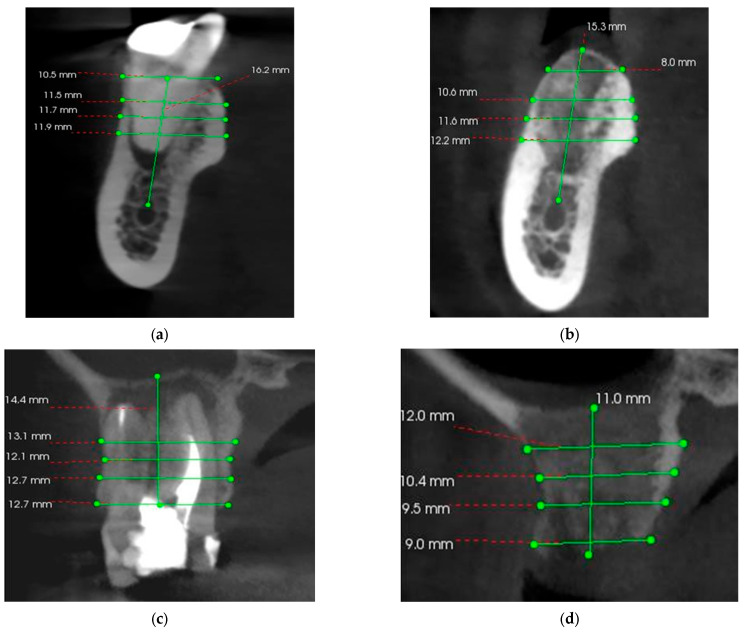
(a) Pre-operative CBCT at the level of the left mandibular first molar (36); (b) 3 months postoperative CBCT at the level of the left mandibular first molar (36); (c) Pre-operative CBCT at the level of the right maxillary first molar (16); (d) 3 months postoperative CBCT at the level of the right maxillary first molar (16).
